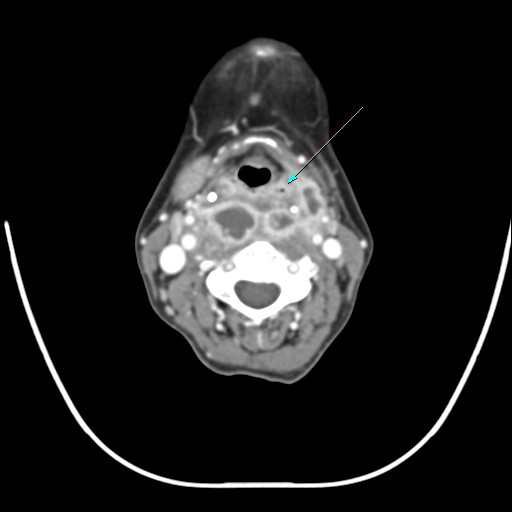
CT scan showing abscess formation beginning around the piriform sinus.
